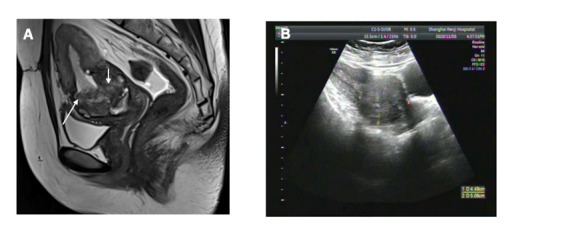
A: MRI showing at T2W sagittal plane a discontinuous lower uterine segment incision (see long white arrow) with the presence of a large mixed soft haemorrhage mass (see short white arrow). B: Ultrasound showing a dilated lower uterine segment with the presence of a heterogeneous mass.
